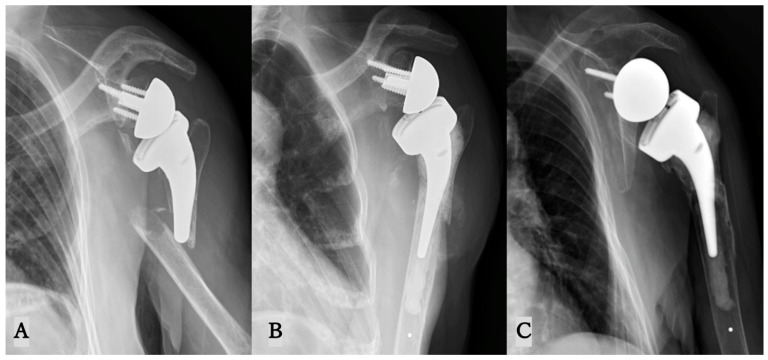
Re-RSA using long-stem conversion and cement fixation. (A): PF of type B1 following RSA. (B): RSA with cemented long stem. (C): Union can be observed on X-ray at the last follow up. PF, periprosthetic humeral fracture. RSA, reverse total shoulder arthroplasty.
